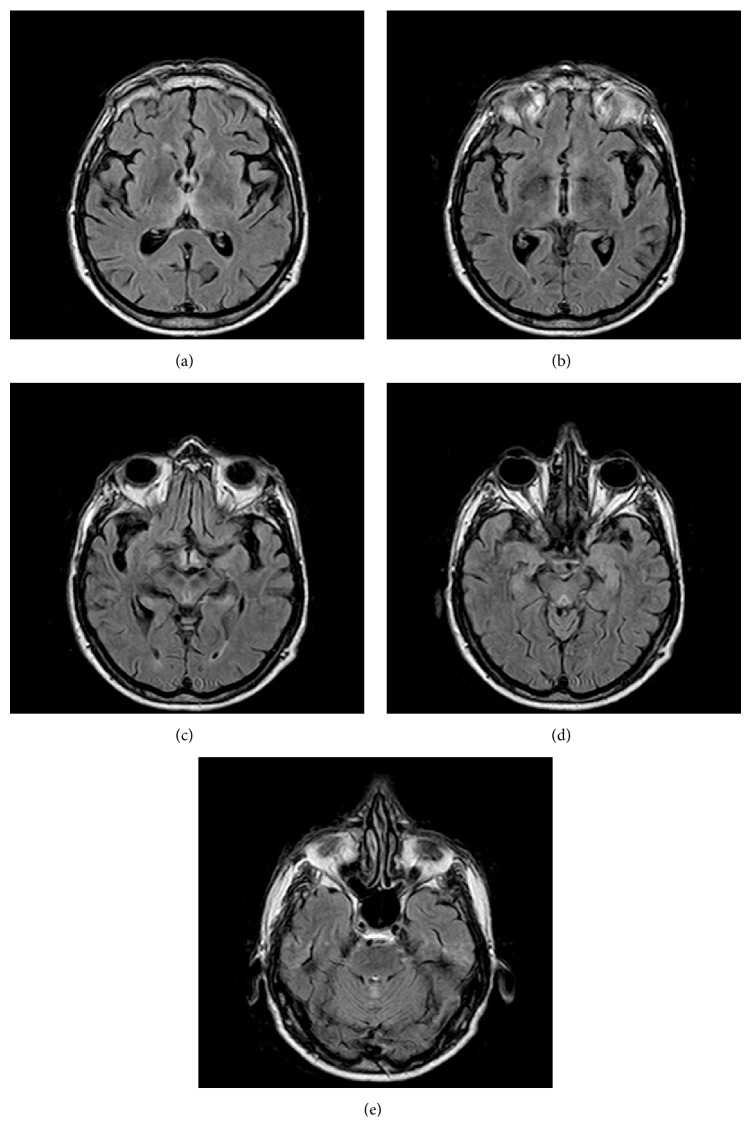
FLAIR axial images of MRI show hyperintensity in (a) the medial region of bilateral thalamus, (b) subependymal grey matter around the third ventricle, (c) third ventricle floor and periaqueductal region, (d) periaqueductal grey matter, and (e) upper cerebellar vermis.
